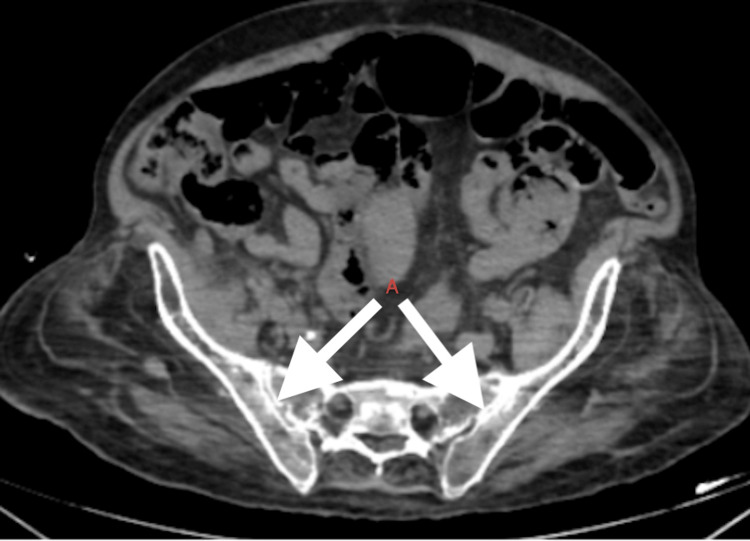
CT scan of bilateral A) sacroiliac joints
